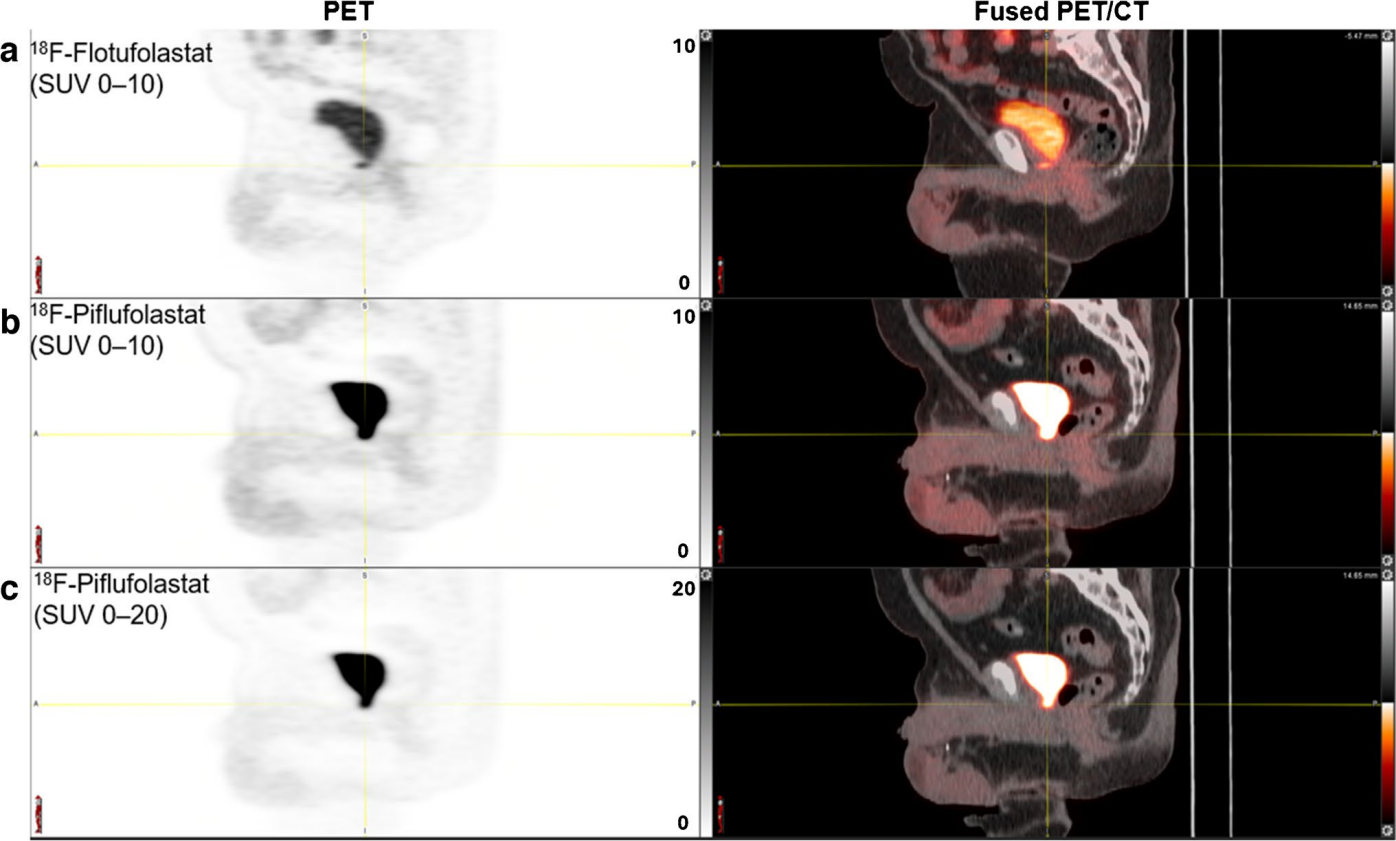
Example PET and fused PET/CT sagittal images from a patient with a baseline PSA level of 0.37 ng/mL who underwent ^18^F-piflufolastat PET and ^18^F-flotufolastat PET one day apart. ^18^F-Flotufolastat PET (row A; SUV scale, 0–10) identified a positive lesion in the vesicourethral anastomosis, which was not identified as separate from the bladder on ^18^F-piflufolastat PET on the same windowing (row B; SUV scale, 0–10), or with wider windowing at SUV 0–20 (row C). The bladder SUV_mean_ was 6.3 with ^18^F-flotufolastat PET and 40.5 with ^18^F-piflufolastat PET. ^18^F=Fluorine-18. CT = computed tomography. PET = positron emission tomography. PSA = prostate-specific antigen. SUV = standardised uptake value
